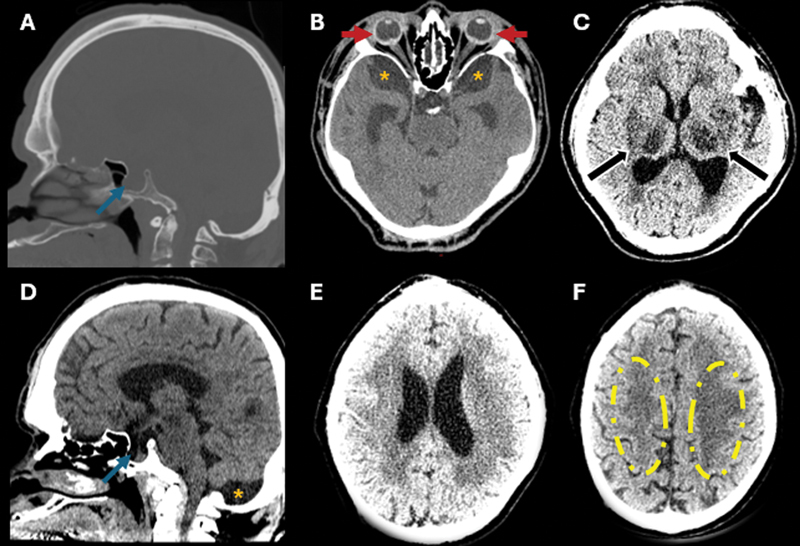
Computed tomography (CT) scan of the head in bone window (
